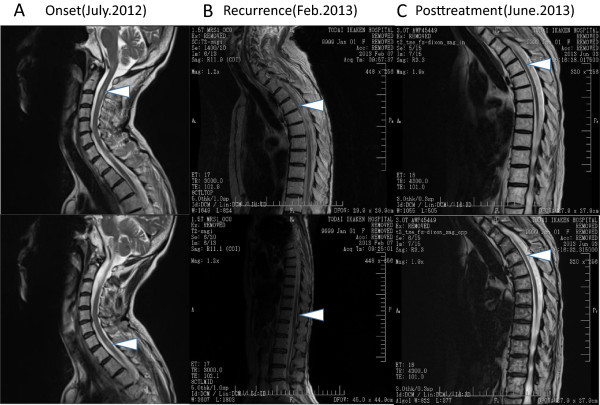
MRI findings. A) At the onset of neurogenic disorder in July 2012. Multiple high-intensity lesions in T2-weighted images (T2WI) of the medulla oblongata, cervical spinal cord, and thoracic spinal cord were revealed. B) Residual high-intensity lesion in Th3-7. C) Following treatment, the residual lesion in the thoracic spinal cord improved.
